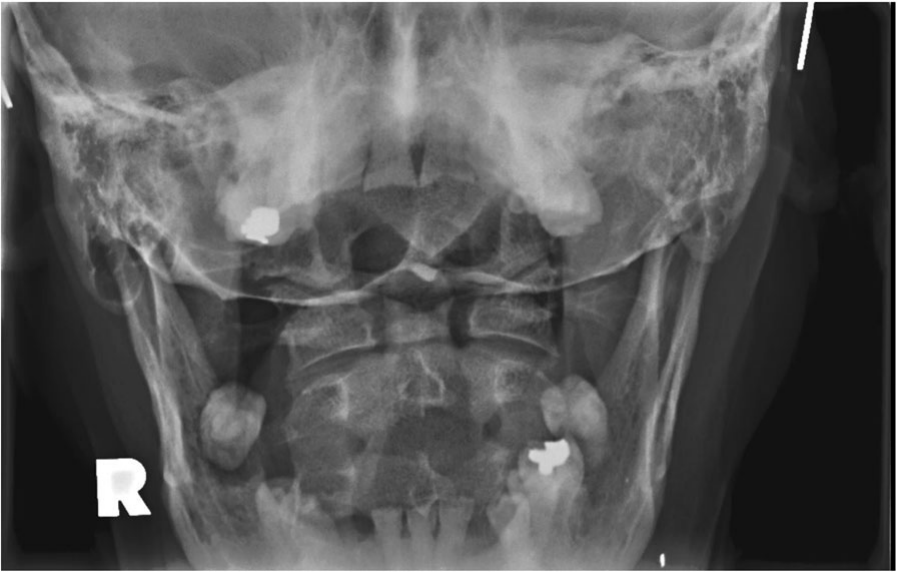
AP Open Mouth Plain Films
